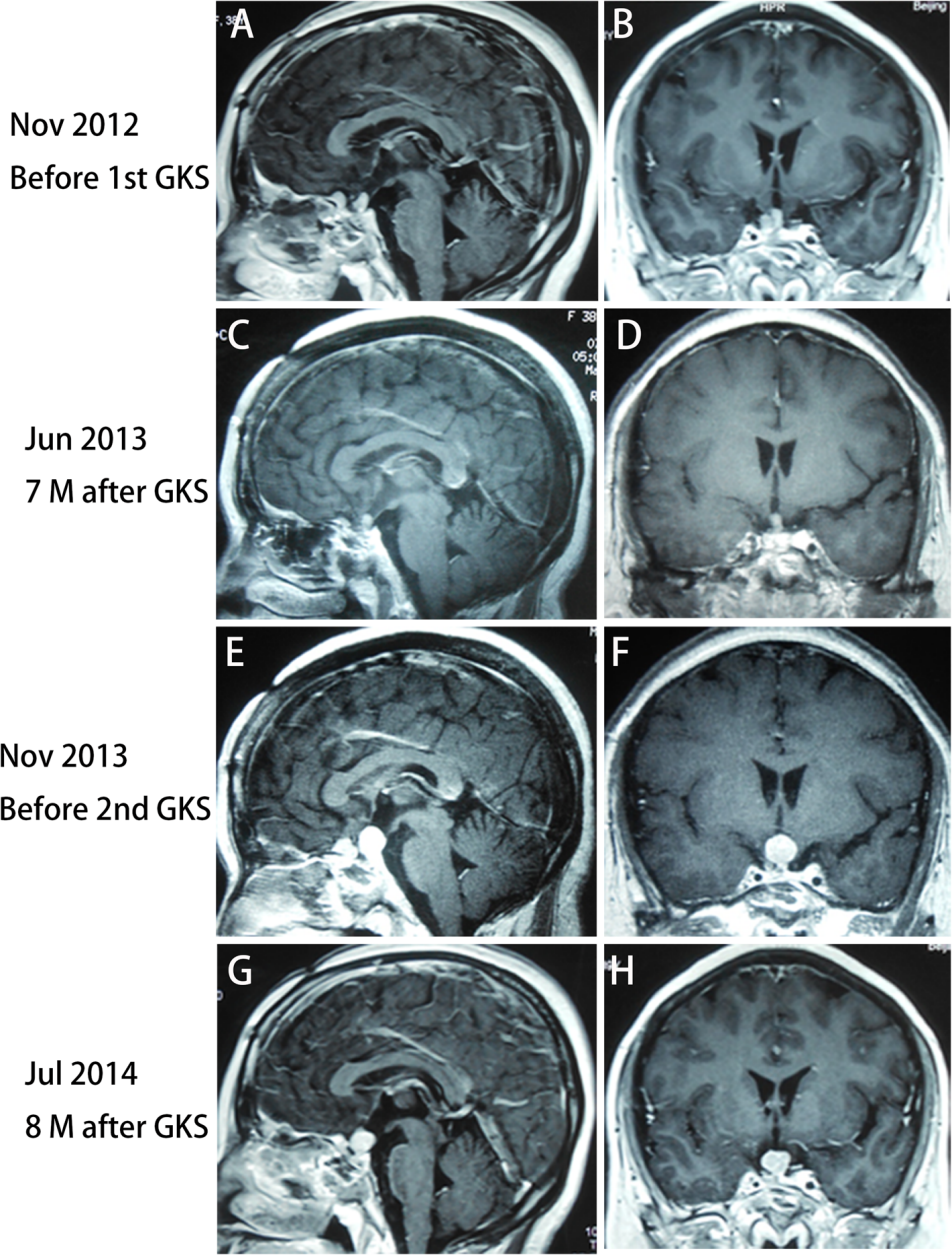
(A) and (B) Before the first gamma knife radiosurgery (GKS) treatment, MRI in November 2012 reported that the tumor was located in the sellar and suprasellar regions. (C) and (D) Seven months after the first GKS treatment, MRI revealed that the tumor size was slightly decreased. (E) and (F) Before the second GKS treatment, MRI in November 2013 demonstrated that the tumor size increased significantly again (Knosp grade 4). (G) and (H) Eight months after the second GKS treatment, MRI in July 2014 indicated that the tumor size was reduced slightly
